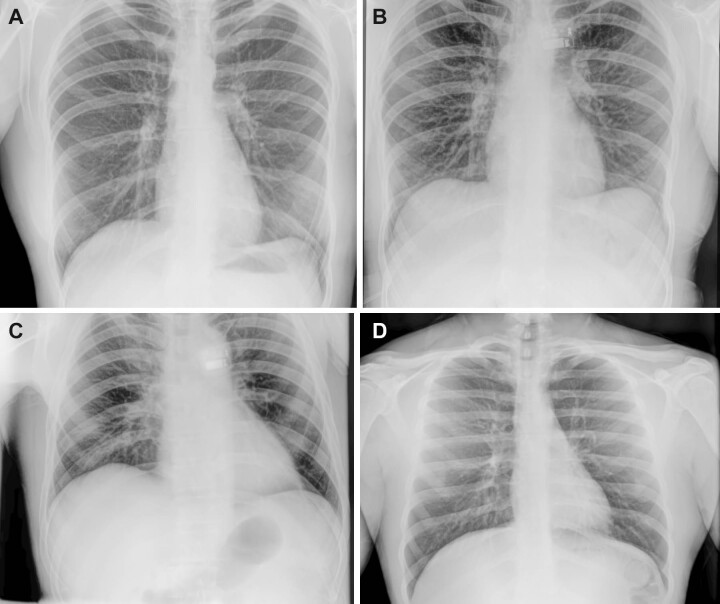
Representative preflight, in-flight, and postflight chest radiographs.
